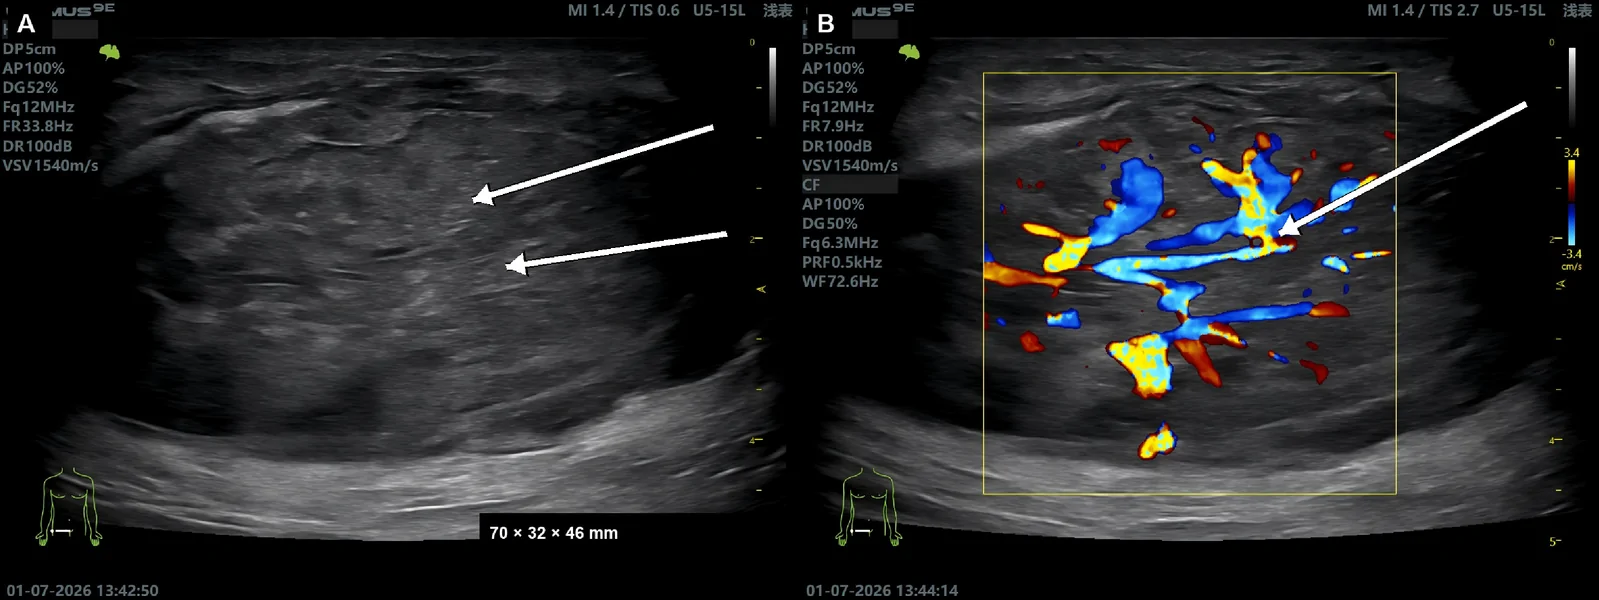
Ultrasonographic findings of the right inguinal region. (A) Gray-scale ultrasonography showed a 70 × 32 × 46 mm heterogeneous hypoechoic mass; white arrows indicate representative internal cord-like hyperechoic bands. (B) Color Doppler imaging showed abundant intralesional flow; the white arrow indicates the central arborizing vascular pattern.
